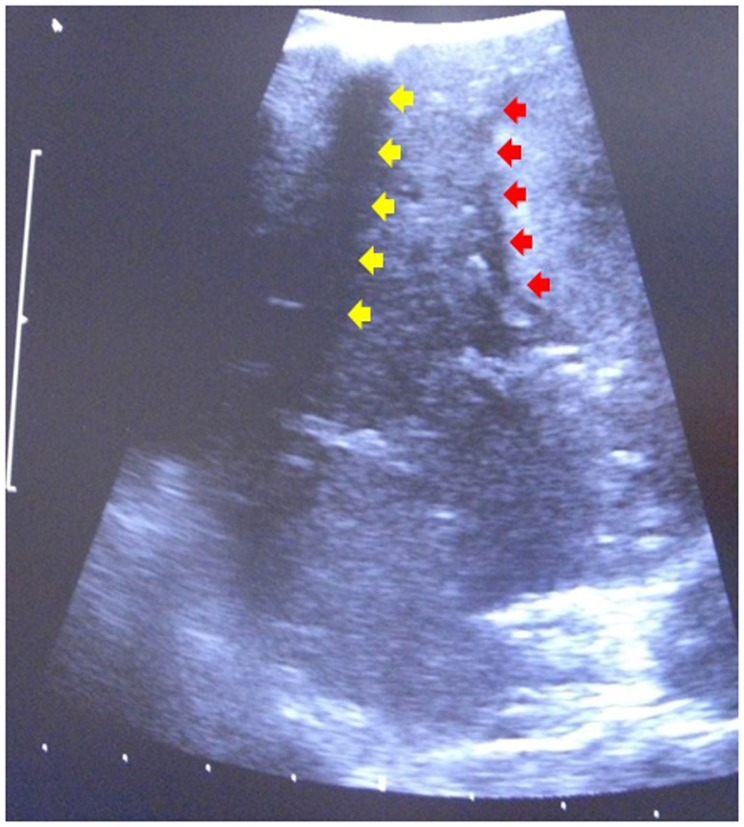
Laparoscopic ultrasound to identify resection margin. Red arrows indicate the tumor margin, yellow arrow indicates a shadow induced by an incision of the liver capsular.
